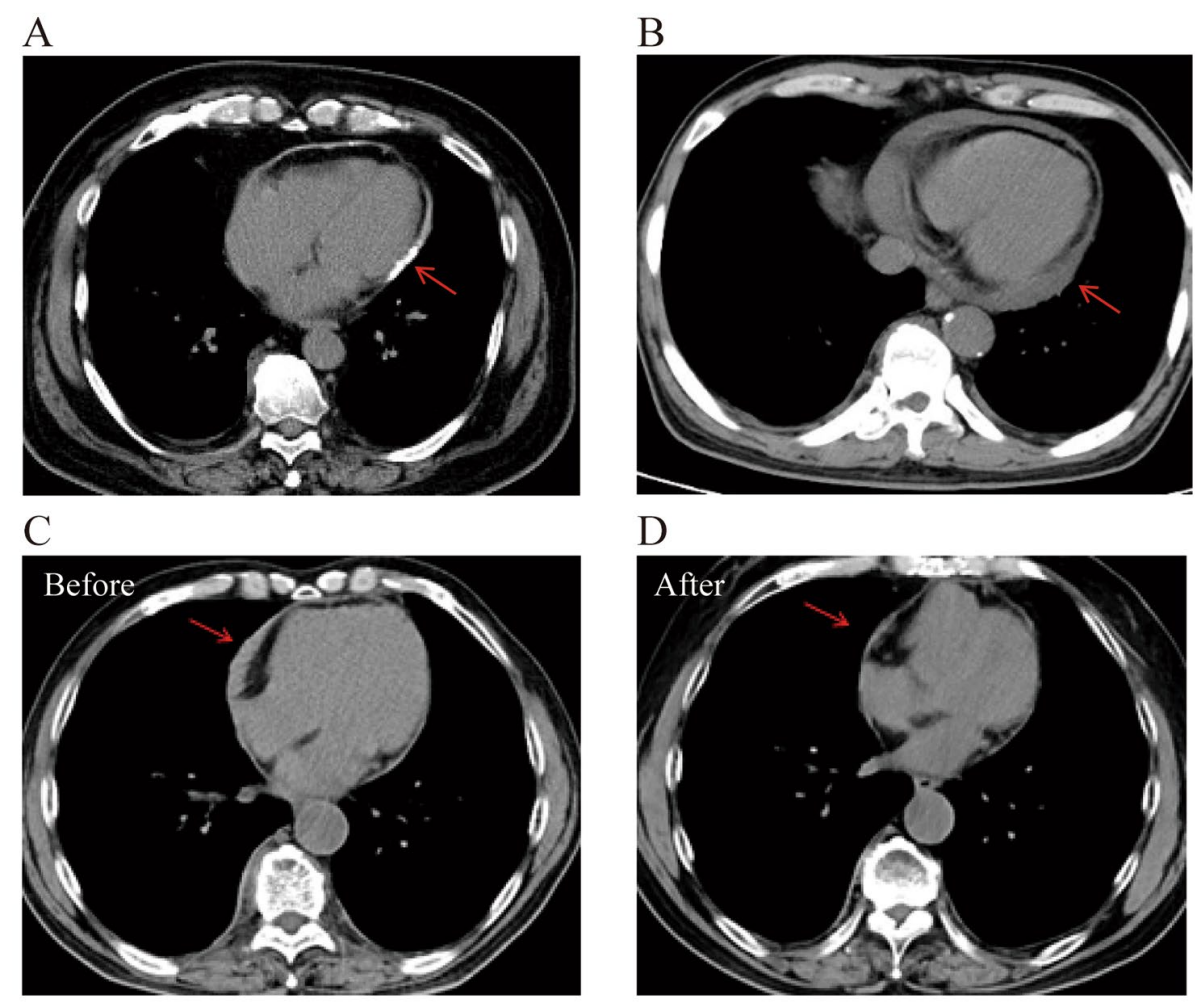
(A) Chest CT revealed pericardial thickening with calcification (arrow). (B) Chest CT indicated pericardial effusion. (C-D) Localized pericardial thickening (arrow) was detected and decreased
